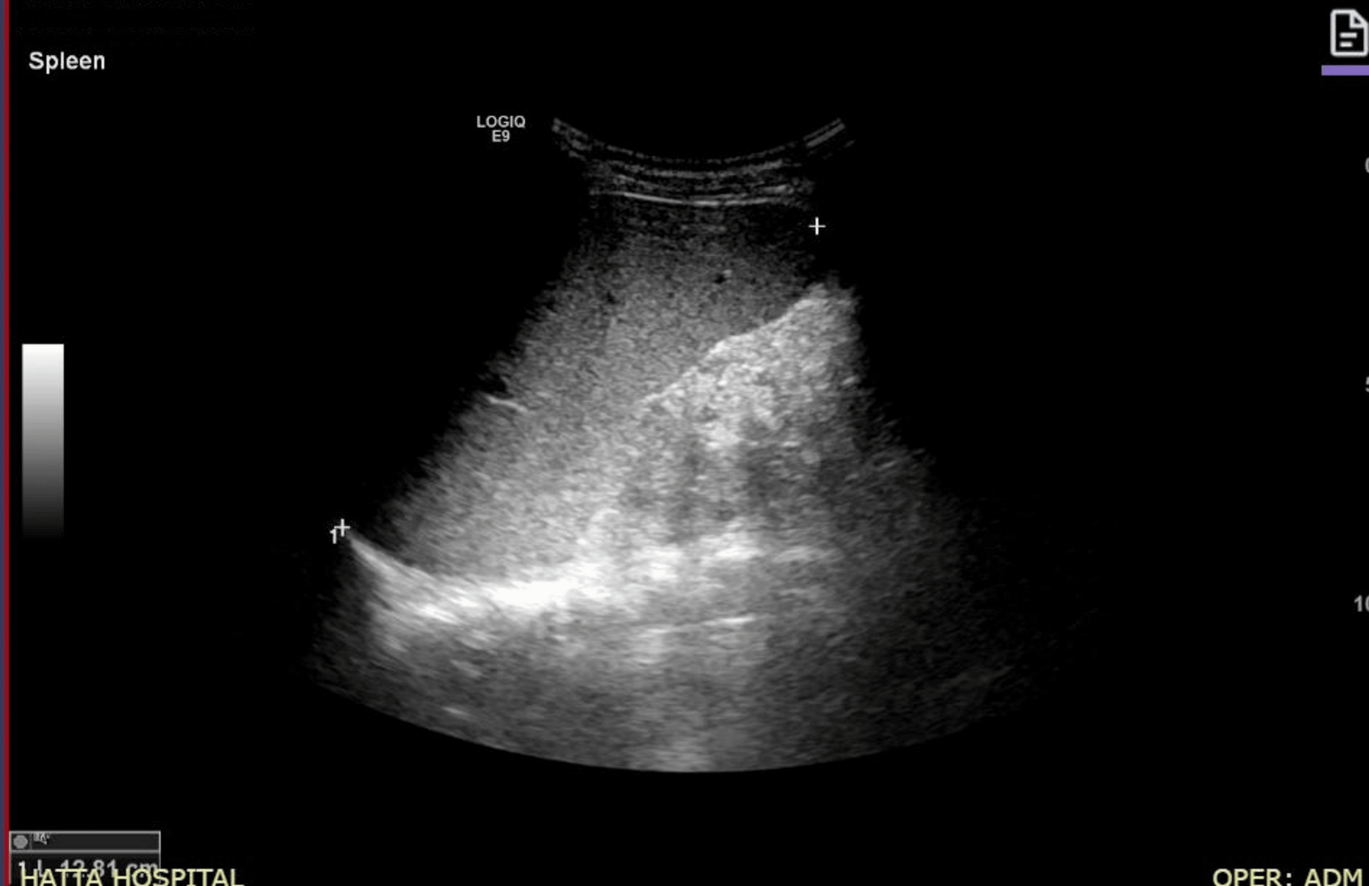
(A) Ultrasonographic Image of the Spleen The ultrasound image demonstrates an enlarged spleen, indicative of splenomegaly.
